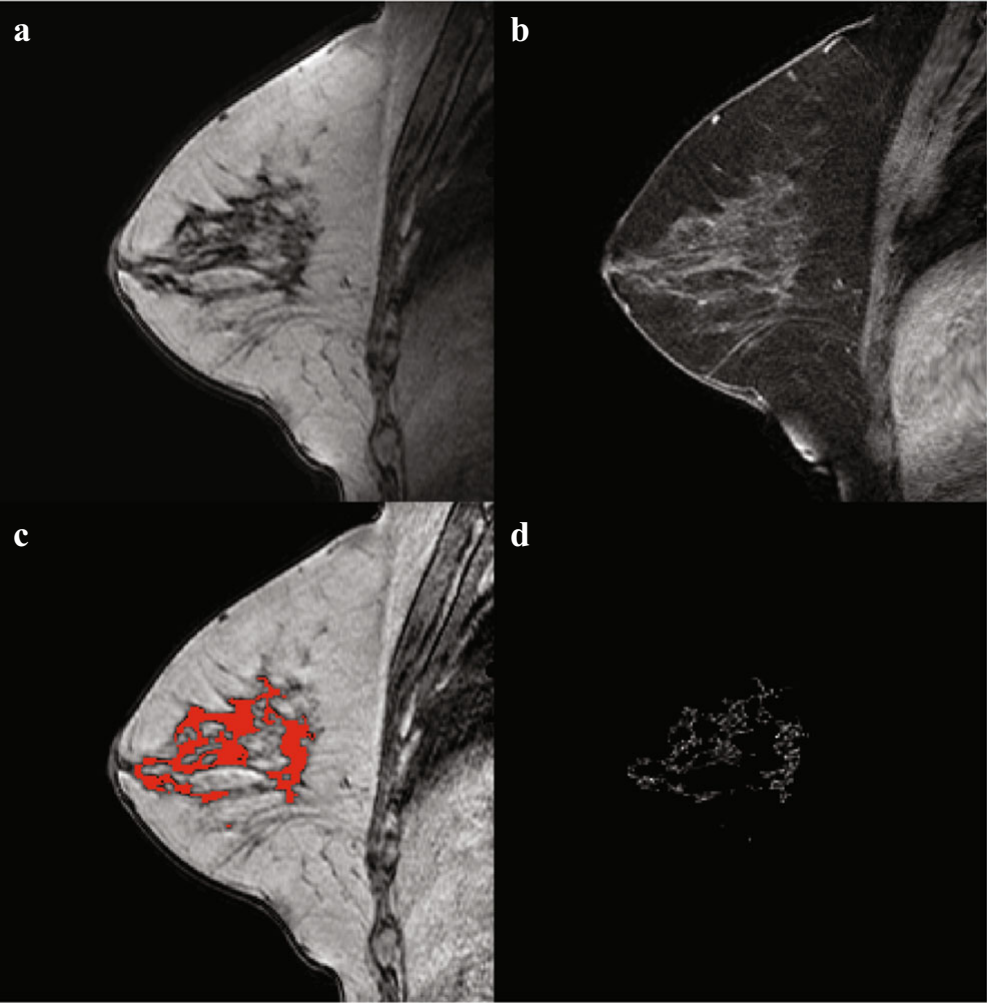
Example of the image processing in the contralateral breast of a 56-year-old patient with ER-positive/HER2-negative cancer patient. a non-fat-suppressed T1-weighted MRI, b fat-suppressed T1-weighted MRI after intravenous administration of contrast, c bias-field corrected image with the parenchymal tissue segmentation overlayed in *red*, d late enhancement in the parenchymal tissue segmentation
